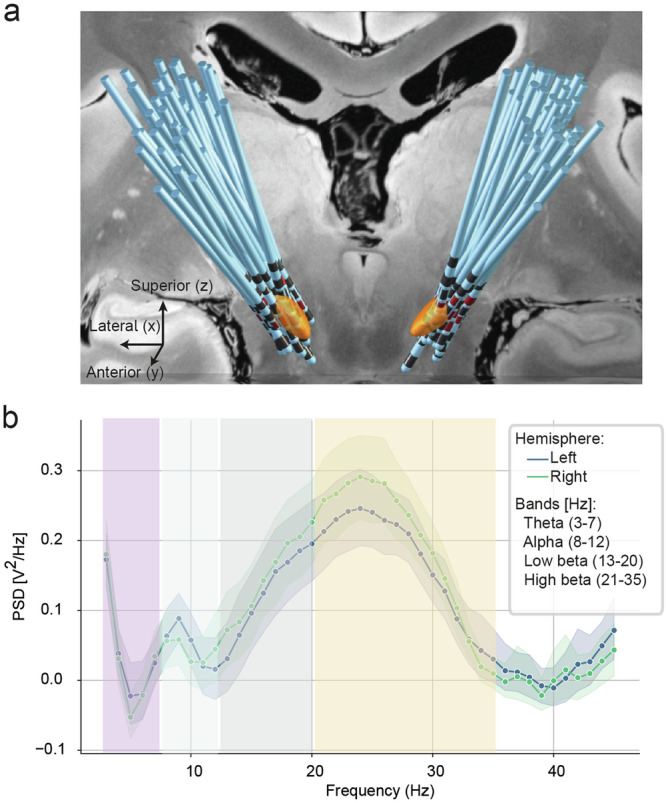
Electrophysiological features and electrode positions. a Reconstructed electrode leads in the subthalamic target region in the Synthesized FLASH25 100 Micron MRI in MNI Space^63^. b PSDs with the aperiodic 1/f component removed were averaged for the left (blue) and right (green) hemispheres across all patients. Additionally, theta, alpha, low beta (13–20 Hz), and upper beta (21–35 Hz) frequencies are shaded in pink, light blue, gray, and orange, respectively. Solid lines represent mean values, whereas shaded areas represent 95% confidence intervals.
